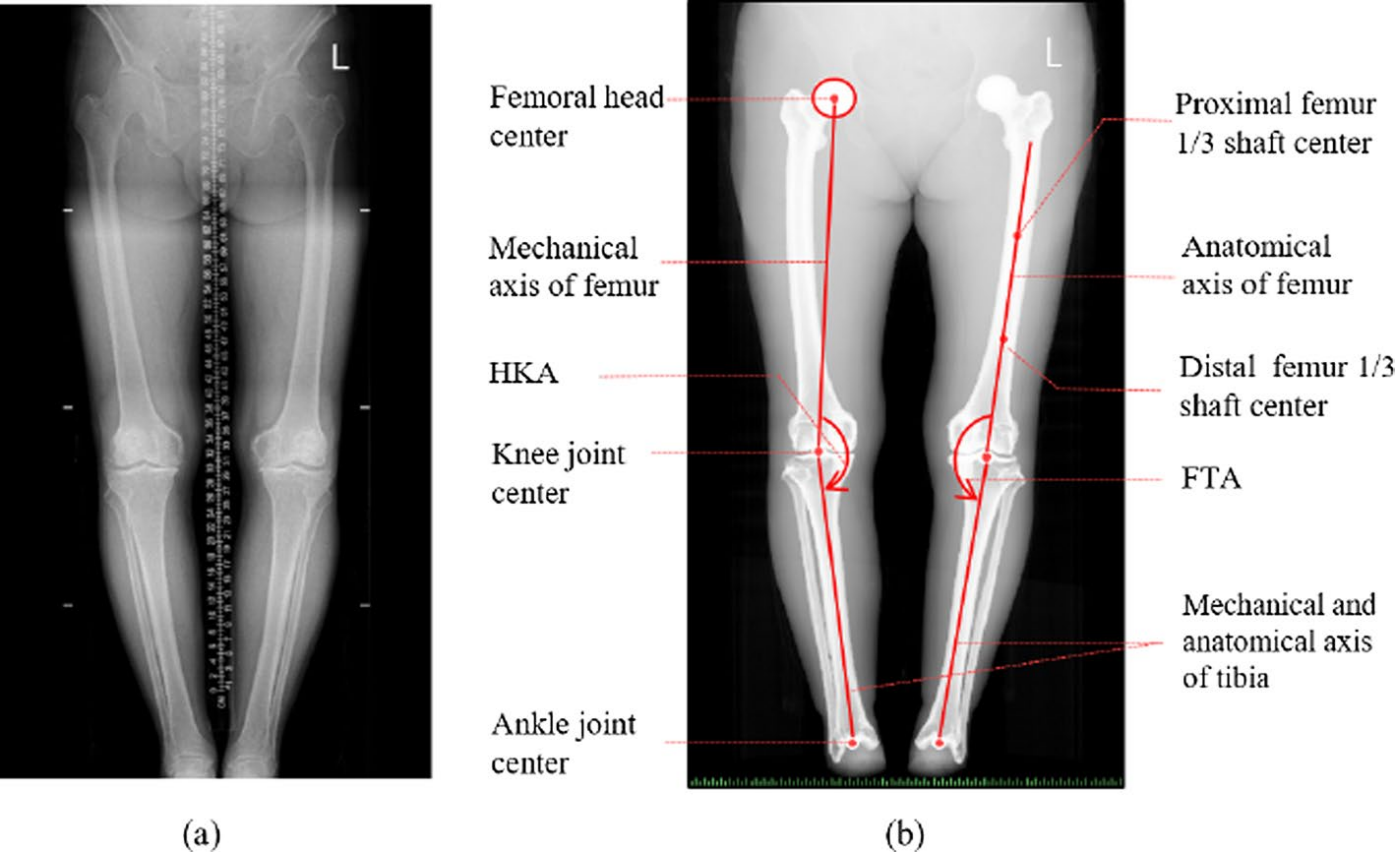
A real long-leg radiograph in clinics and a virtual long-leg radiograph in this study. a The real long-leg radiograph was captured in weight-bearing condition. b The virtual long-leg radiograph was captured in the non-weight-bearing condition. Both mechanical axis and anatomical axis were measured on virtual LLR. The HKA was defined as the mechanical axial of the femur and tibia. The FTA was defined as the anatomical axial of the femur and tibia
